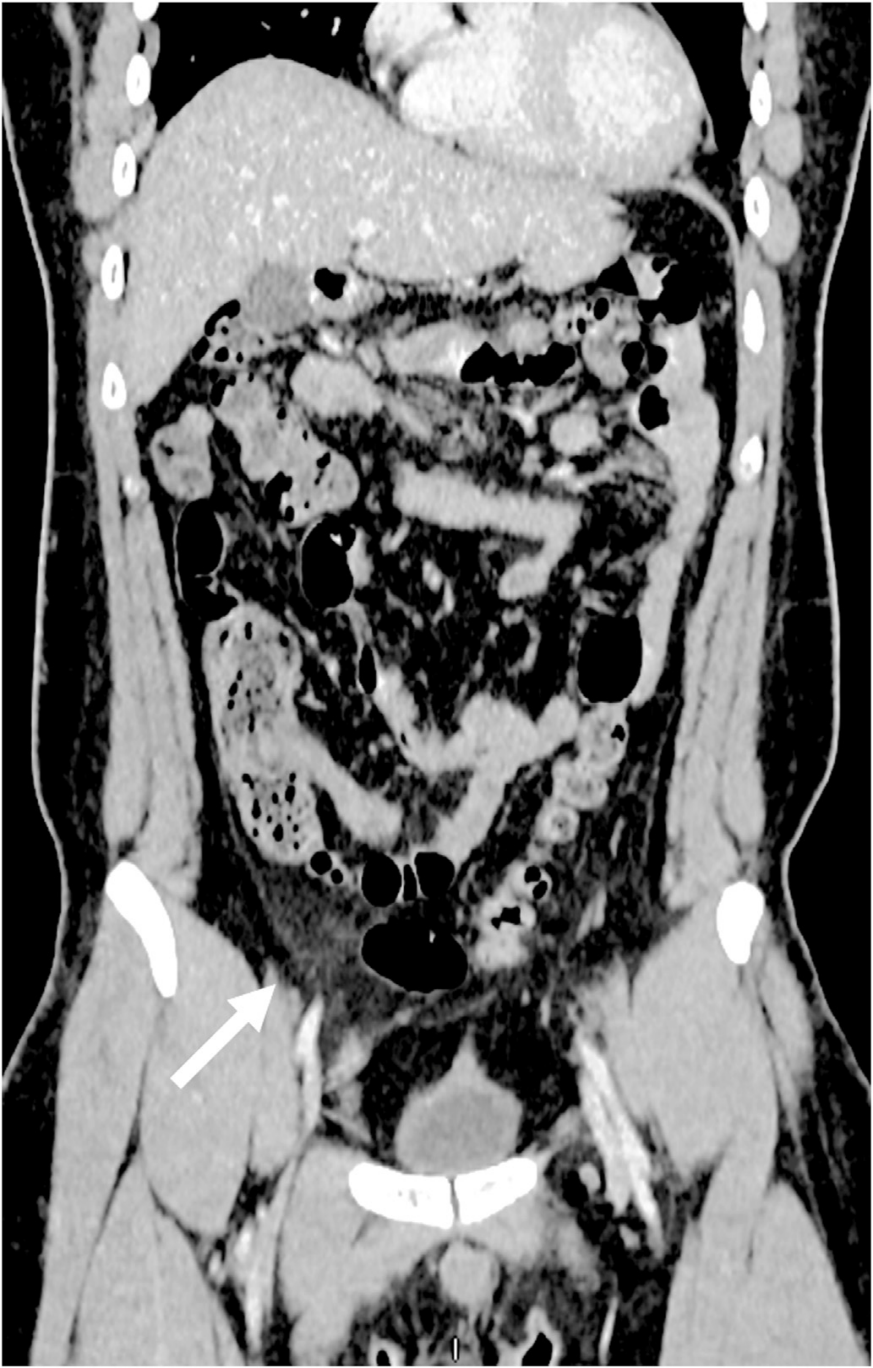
A gastrointestinal representation of COVID-19 demonstrated by computed tomography of the abdomen with arrow highlighting stranding of the mesentery in the abdomen.
